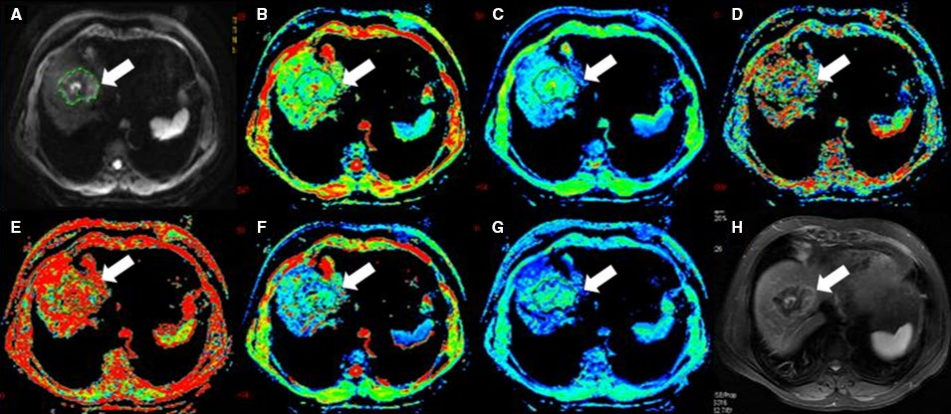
Seventy‐two‐year‐old female with liver metastases(arrow). (A) is b value of 50 s/mm^2^ of DWI, and (B‐G) are pseudocolor of ADC,* D*
